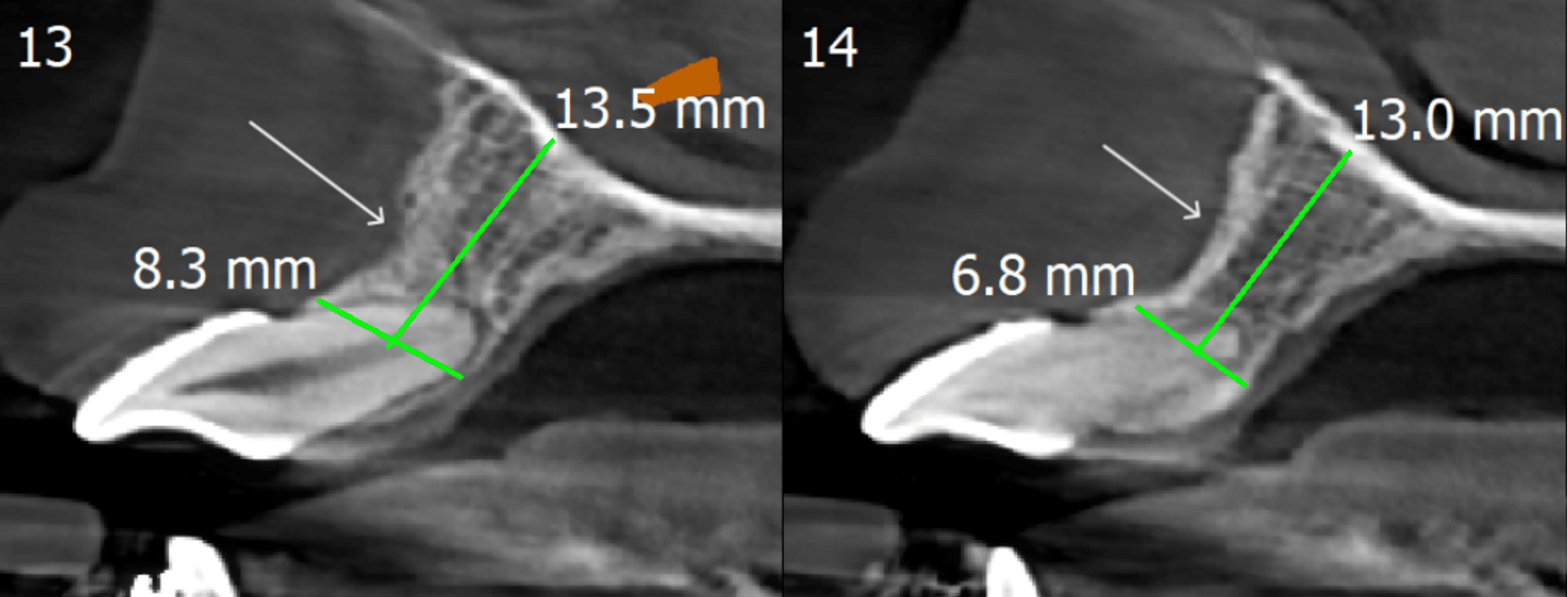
CBCT slide showing labioversion of incisors with compromised buccal plate of the bone. CBCT: cone-beam computed tomography
